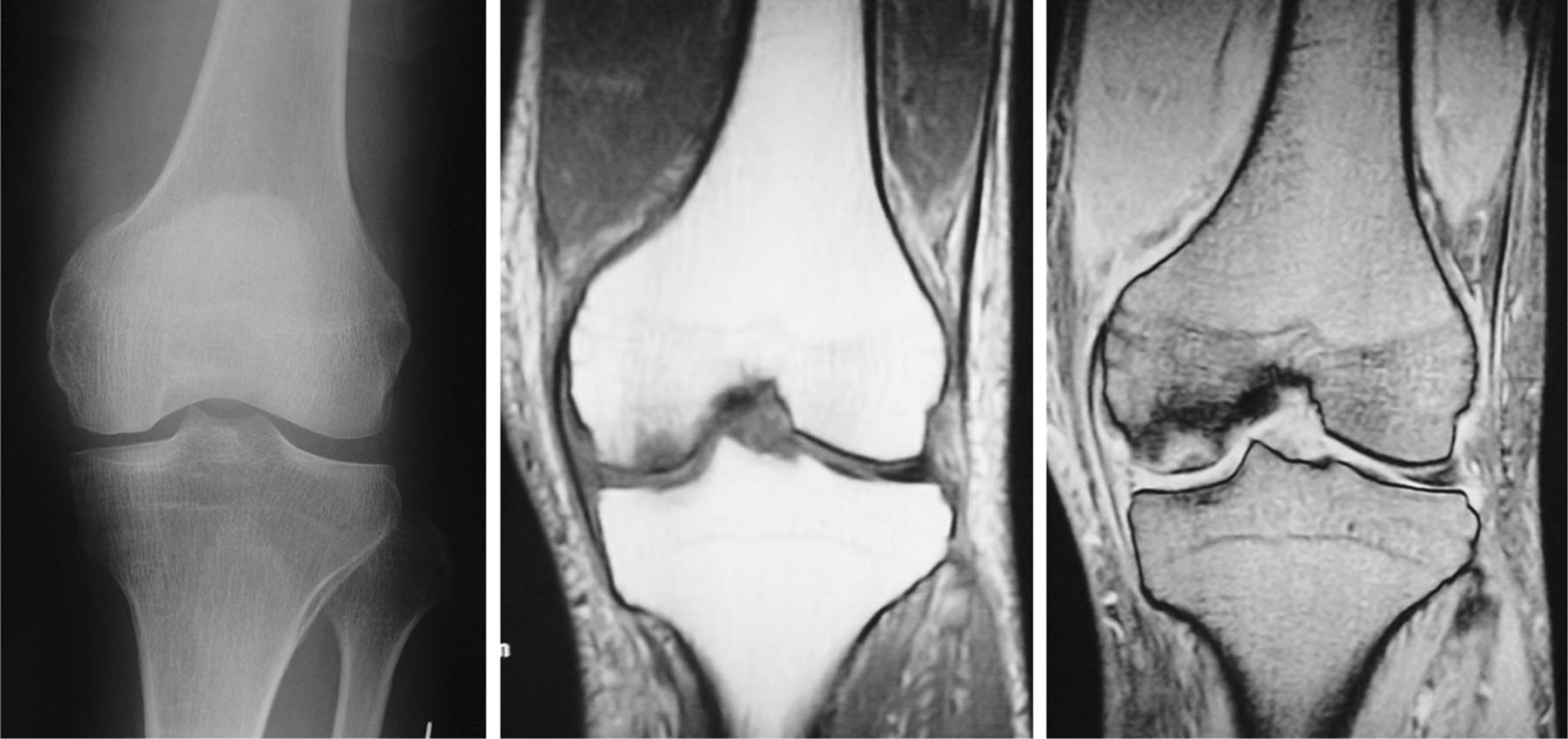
Subchondral insufficiency fracture on plain radiographs and magnetic resonance imaging of a 64‐year‐old male patient.
